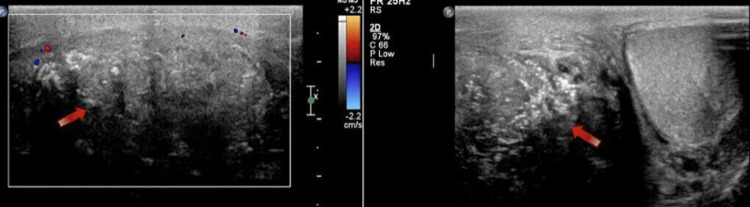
Color Doppler ultrasound showing disrupted, heterogenous right testis in the lateral view (left) and anterior view (right)
